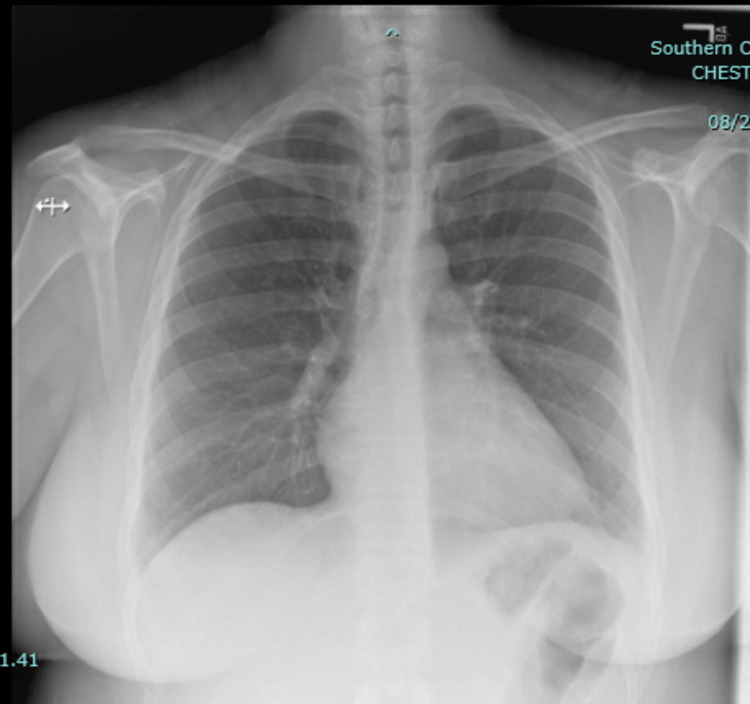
Chest X-ray showing normal lungs, with no consolidation or pleural effusion and normal cardiac silhouette.
